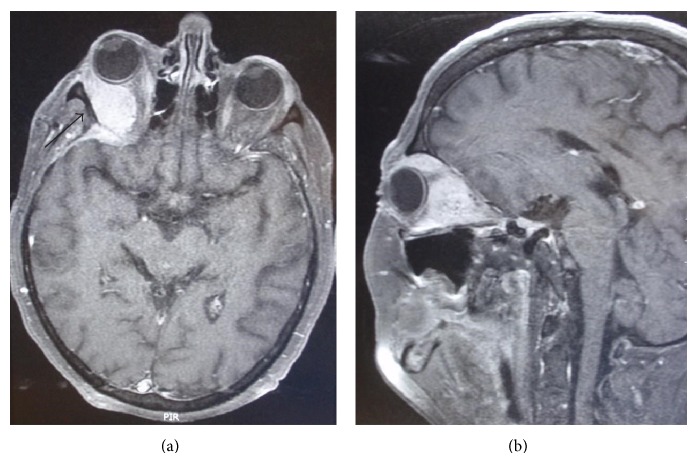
MRI: (a) T2-weighted MRI image of the head showing intraorbital mass and scar tissue (arrow) from previous operation. (b) On contrast T1-weighted image, the intraorbital mass may be clearly seen, as well as exophthalmos.
